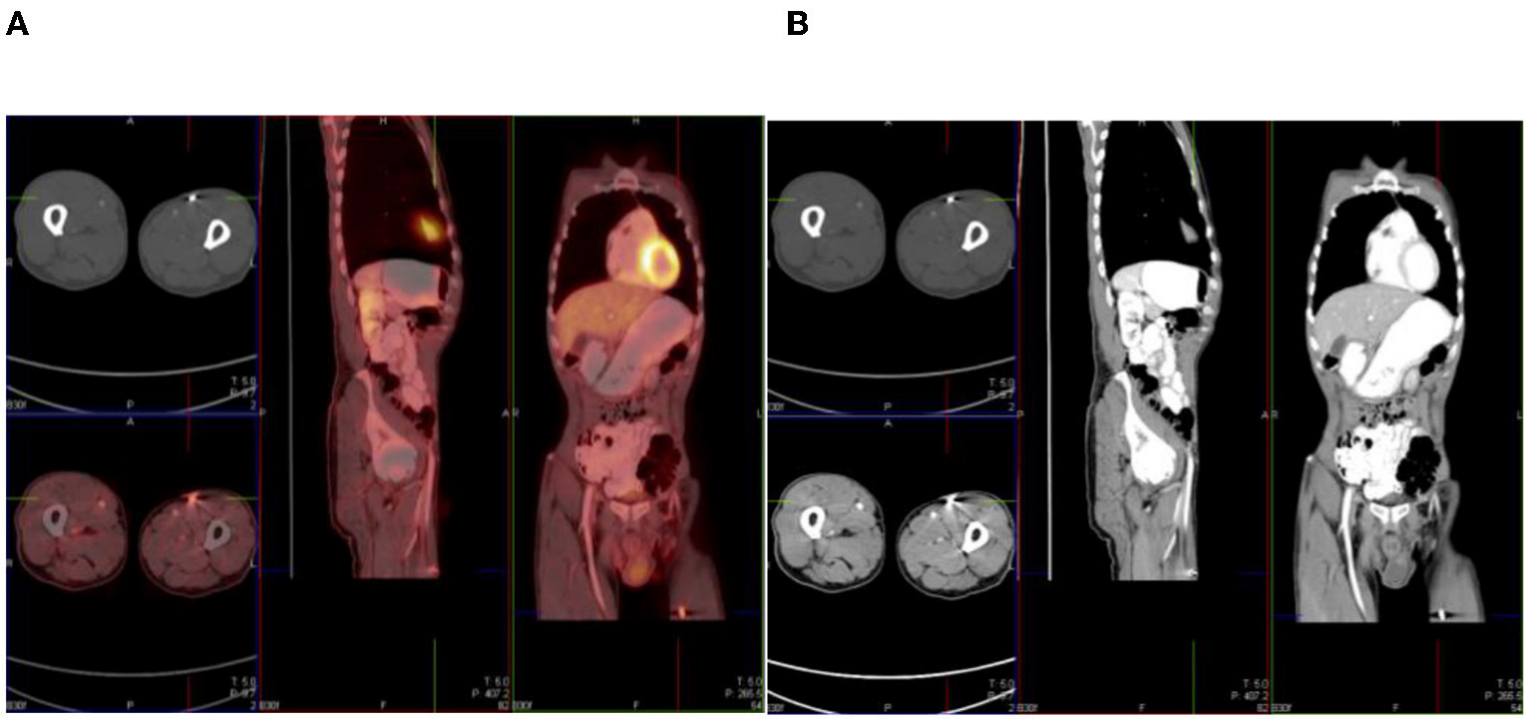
A 56-year-old man with sinonasal carcinoma of the hard palate. Combined PET/CT (A) and CT (B) images show intense uptake related to a metallic artifact in the left anterior thigh.
